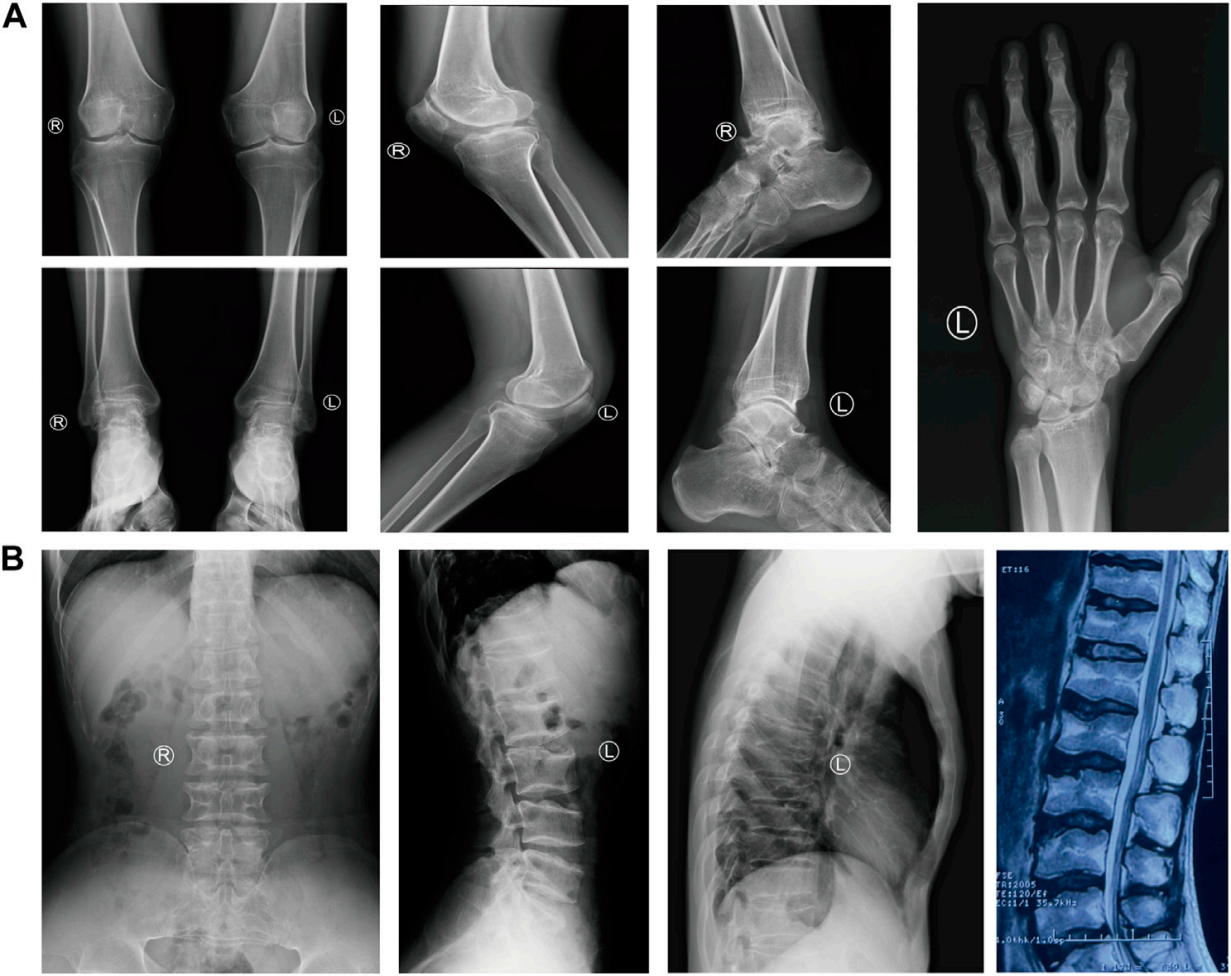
The radiological findings of osteoarthritis in the proband. (A) Radiographs of the knees, ankles, left hand and foot, showing the diffuse joint-space-narrowing. (B) Radiographs and MRI of the spine, showing platyspondyly.
